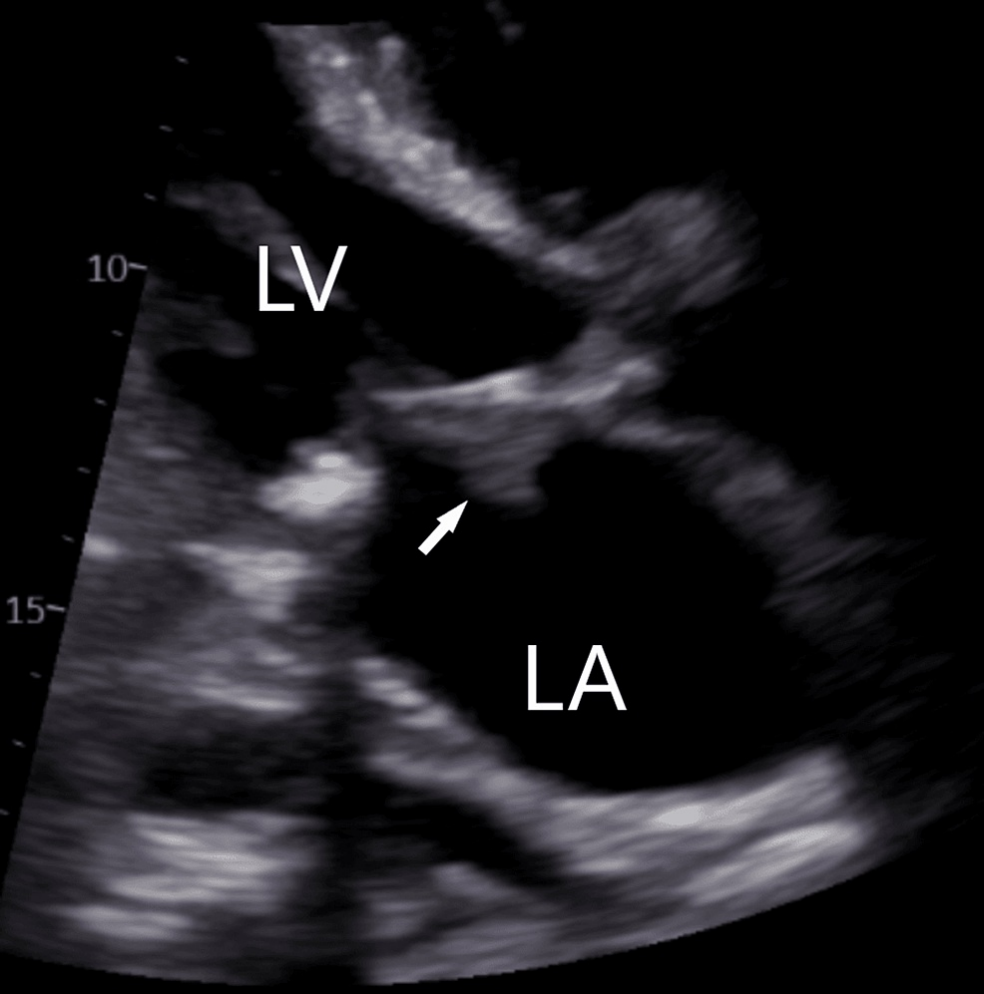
TTE showing an irregular and mobile vegetation measuring 17 mm x 14 mm on the body of the anterior leaflet (arrow). LA, left atrium; LV, left ventricle; TTE, transthoracic echocardiogram
